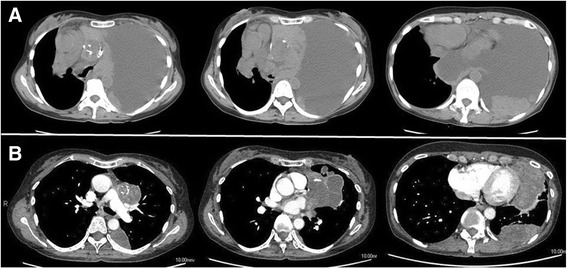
a Chest CT scan showing an anterior mediastinal tumor with abundant left pleural effusion and multiple pleural tumors. b CT scan image obtained after chemical pleurodesis and systemic chemotherapy showing a mediastinal tumor that has invaded the hilum of the left lung and multiple disseminated masses
